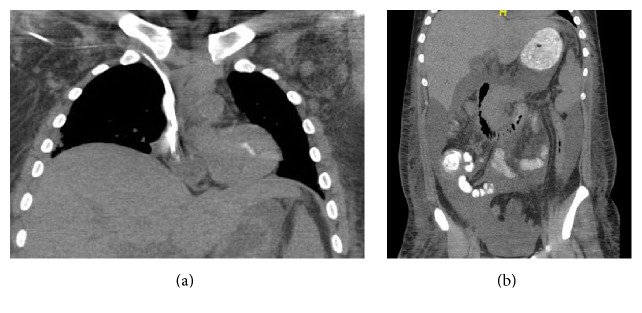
Computed tomography demonstrating diffuse axillary lymphadenopathy measuring 1 to 1.2 cm in short axis (a) and ascites (b).
